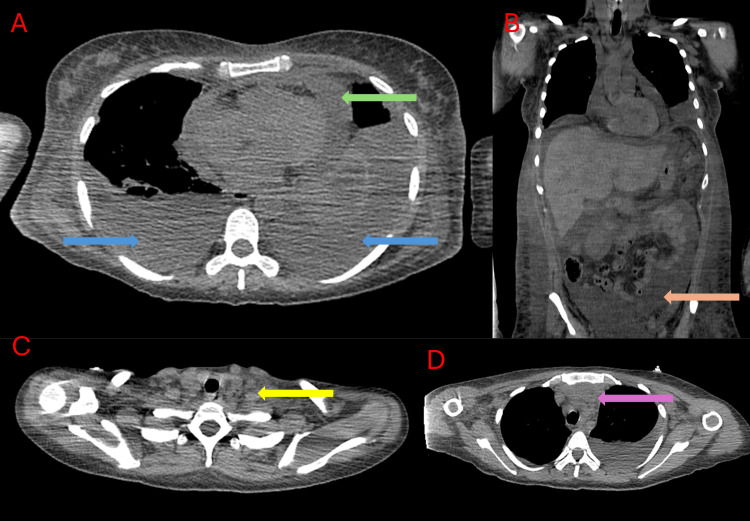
Computed tomography (CT) scan captured at admission. (A) Chest CT image showing bilateral pleural effusion (blue arrows) and pericardial effusion (green arrow). (B) Abdominal CT image showing ascites (orange arrow). (C) Cervical CT image showing multiple cervical lymphadenopathies (yellow arrow). (D) Chest CT image showing a poorly defined prevascular mediastinal mass (purple arrow).
